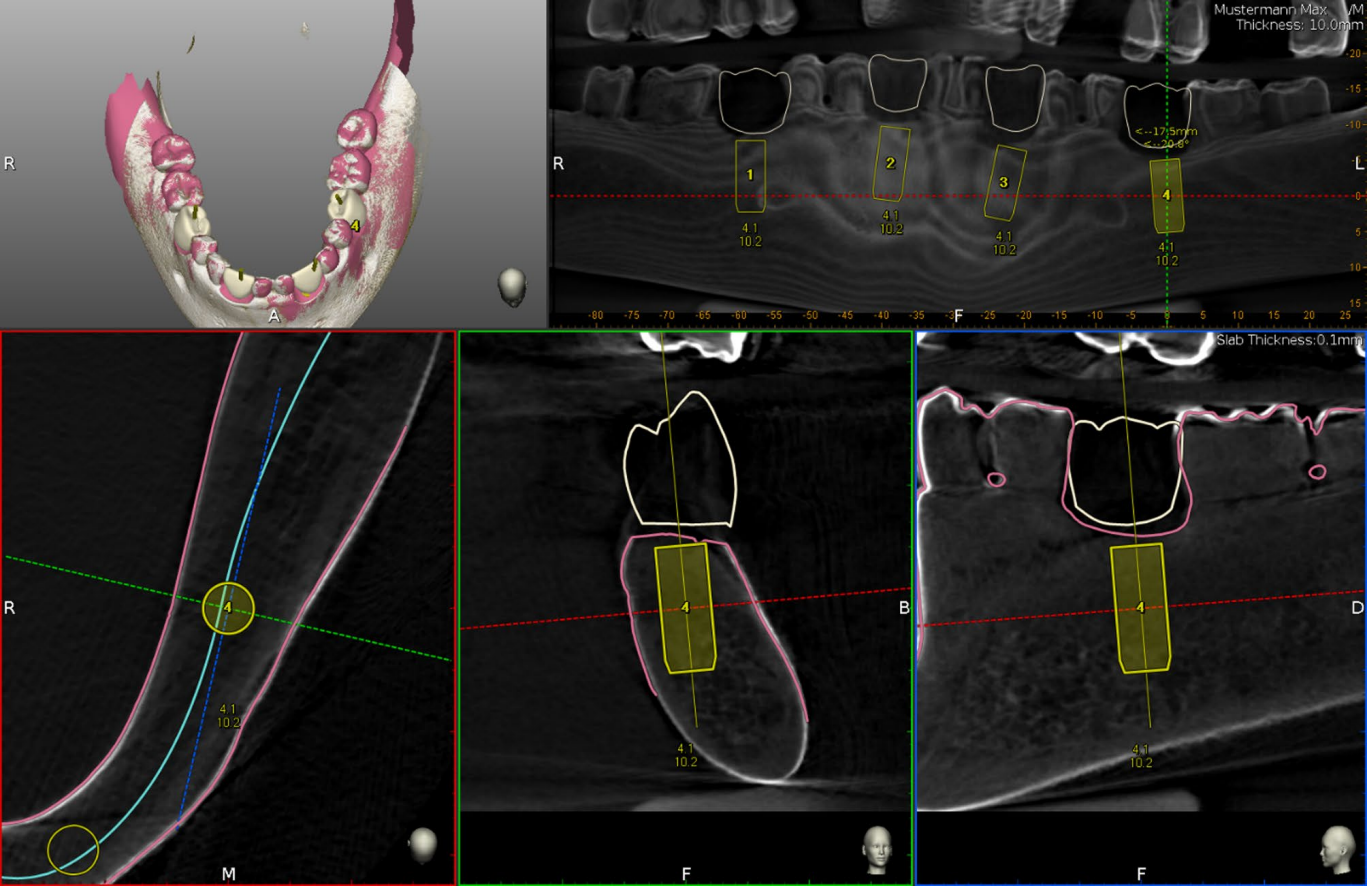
Exemplary digital implant planning in the IPS of the investigated dCAIS system. Abbreviations: IPS (implant planning software), dCAIS (dynamic computer-assisted implant surgery), CBCT (cone-beam computed tomography); grey shades: CBCT data, yellow: virtually planned implants (4.1 × 10 mm), white: digital wax-ups, pink: scan data
